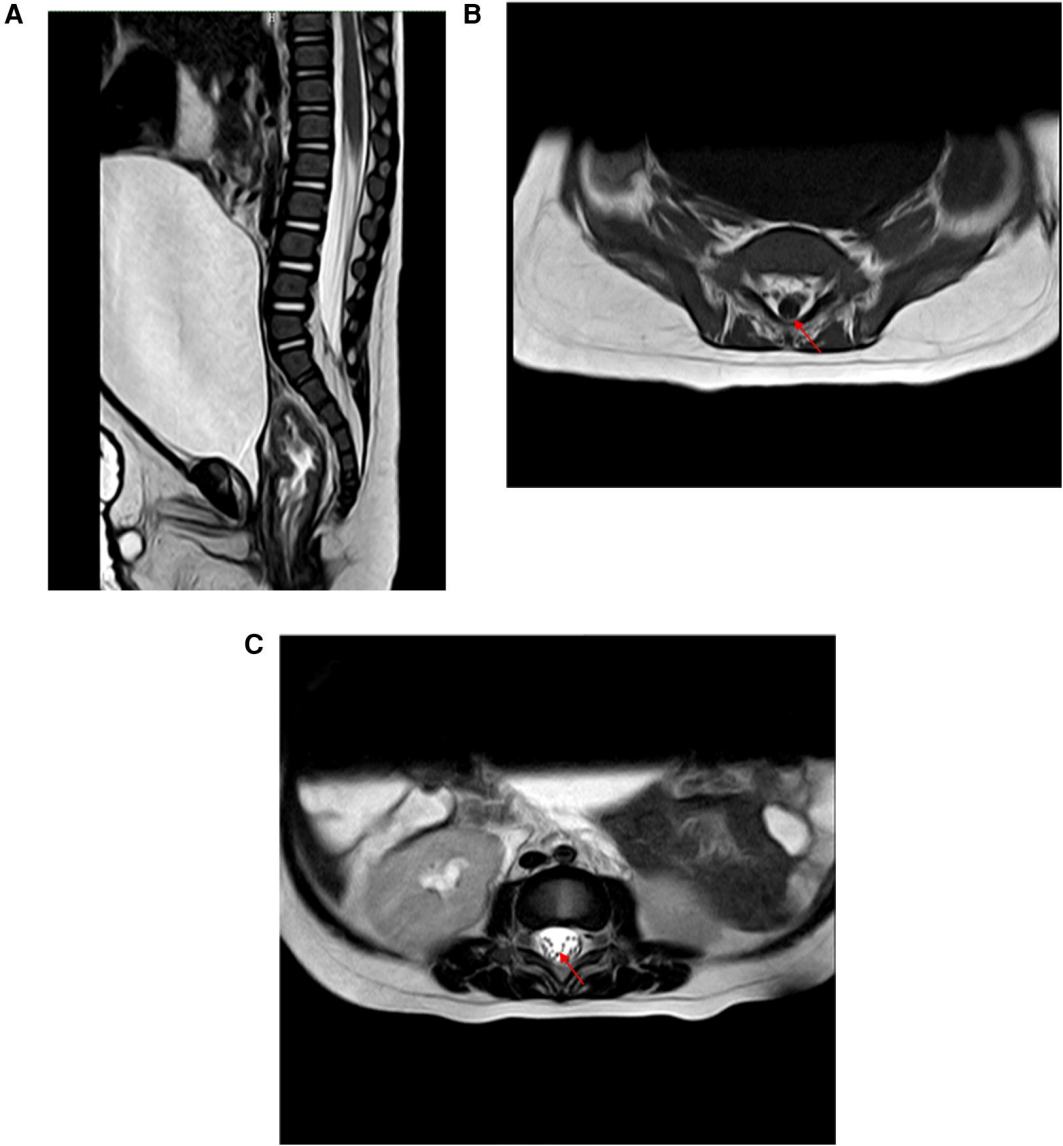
(A) MRI revealed bladder distension. (B,C) MRI revealed fatty degeneration of the filum terminale (red arrow).
